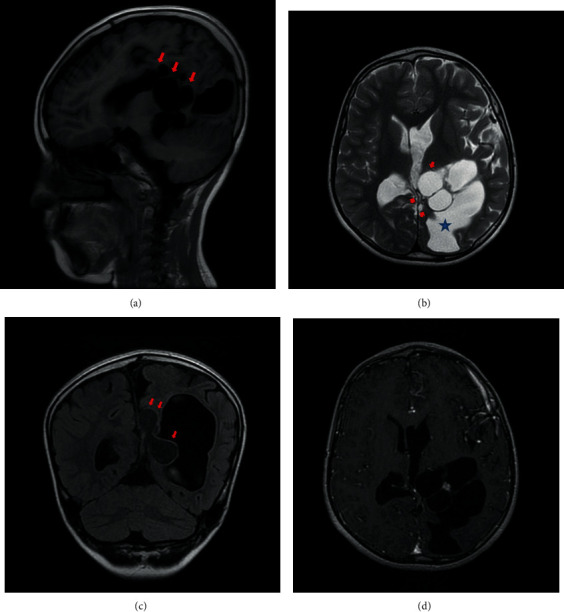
(a) Sagittal slice of T1-weighted image showing the cyst in low signal (arrows). (b) Axial slice of T2-weighted image showing high-signal cyst (arrows) compressing the interventricular foramen responsible for a ventriculomegaly (star). (c) Coronal slice of T2-flair image showing a discreet high signal of the cyst (arrows) compared to the CSF. (d) Axial slice T1-weighted image after gadolinium injection showing the absence of enhancement of the cyst (arrows).
